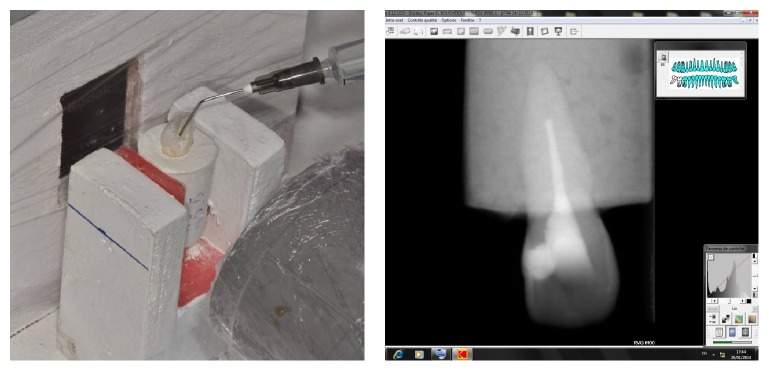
Passive irrigation with conventional needle and the corresponding radiograph.
